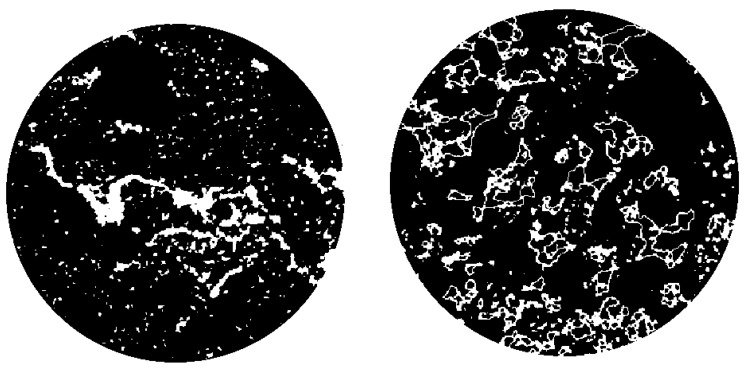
X-ray CT images of 0% (left) and 1% (right) CB content clay bricks.
